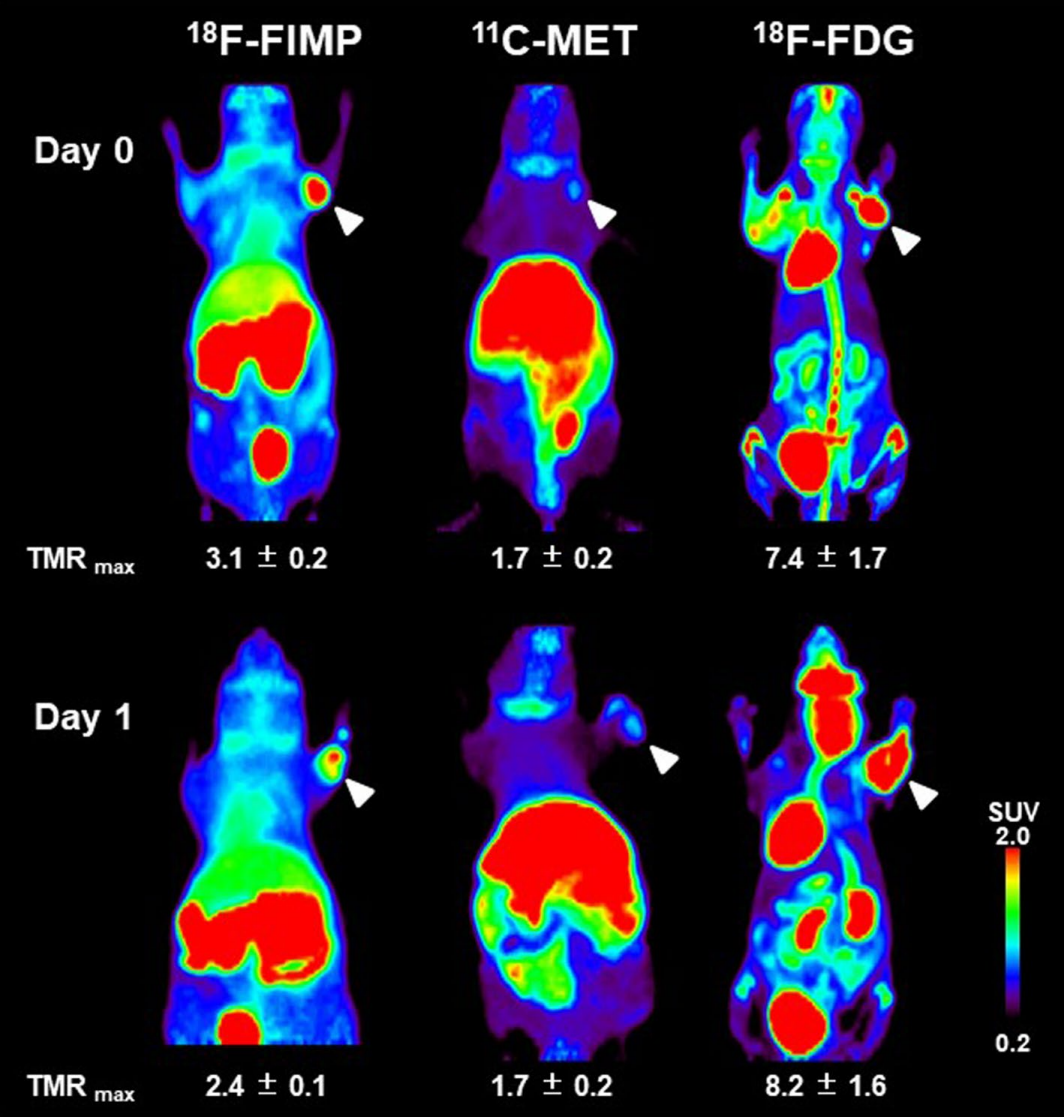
PET probe accumulation in LNZ308 tumors after irradiation. Images shown are the maximum intensity projection (MIP) image of [^18^F]FIMP, [^11^C]MET, and [^18^F]FDG-PET after irradiation on days 0 and 1. Tumors on right paws, as indicated by arrows, were irradiated on day 0 at the dose of 60 Gy. PET data were acquired 90 min after injection of [^18^F]FIMP and [^11^C]MET. [^18^F]FDG-PET data was acquired 55 to 100 min after injection. Data were reconstructed using OS-EM algorithm. Part of this figure was sourced from the following paper. Sci Rep. 2019 Oct 31;9(1):15718. 10.1038/s41598-019-52270-x.
